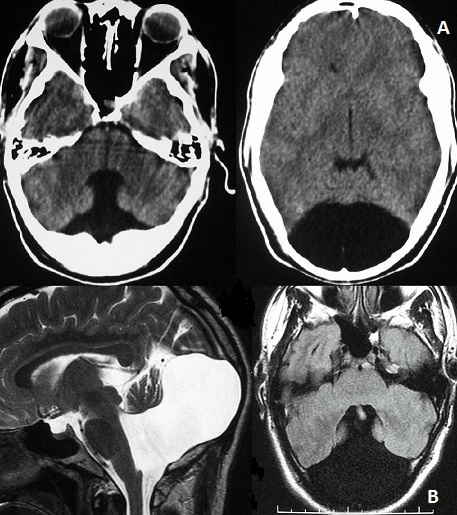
(A) CT scan showing agenesis of cerebellar vermis, large posterior fossa cyst communicating with the fourth ventricle suggestive of Dandy-Walker malformation (left) and thinning of the occipital bone (right). (B): magnetic resonance imaging, T2 sagittal view (left) and T2 Flair axial view (right), showing agenesis of cerebellar vermis and large cerebrospinal-fluid filled cyst in the posterior fossa that is confluent with the fourth ventricle without hydrocephalus
